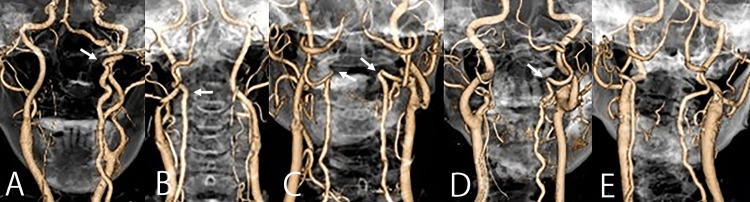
Preoperative three-dimensional CT angiography showing anterior and posterior views with bone-transparent cervical spine reconstruction. (A) Case 1, (B) Case 2, (C) Case 3, (D) Case 4, and (E) Case 5. White arrows: HRVA. HRVA: high-riding vertebral artery; CT: computed tomography.
